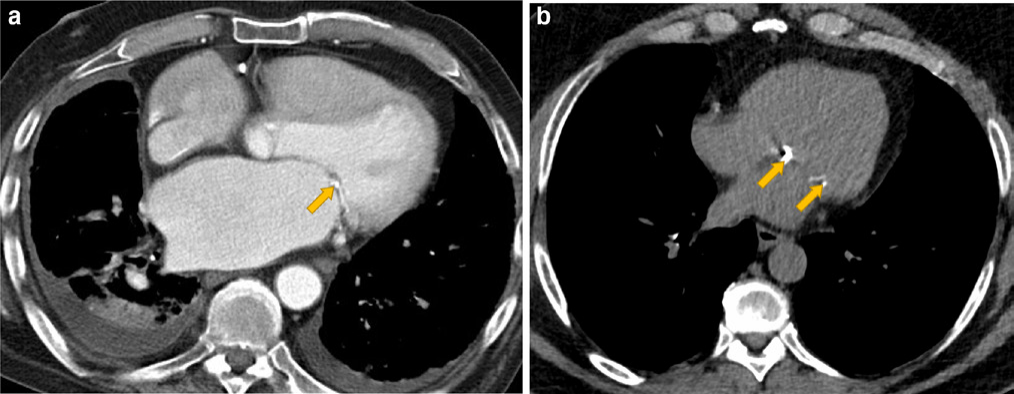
Mitral leaflet and mitral annular calcification. Contrast enhanced CT (A) shows mitral leaflet calcification (A, yellow arrow) in a patient with mitral valve dysfunction and associated enlarged left atrium and pleural effusions. In comparison the non-contrast CT (B) shows mitral annular calcification (yellow arrows) without clinical sequelae.
